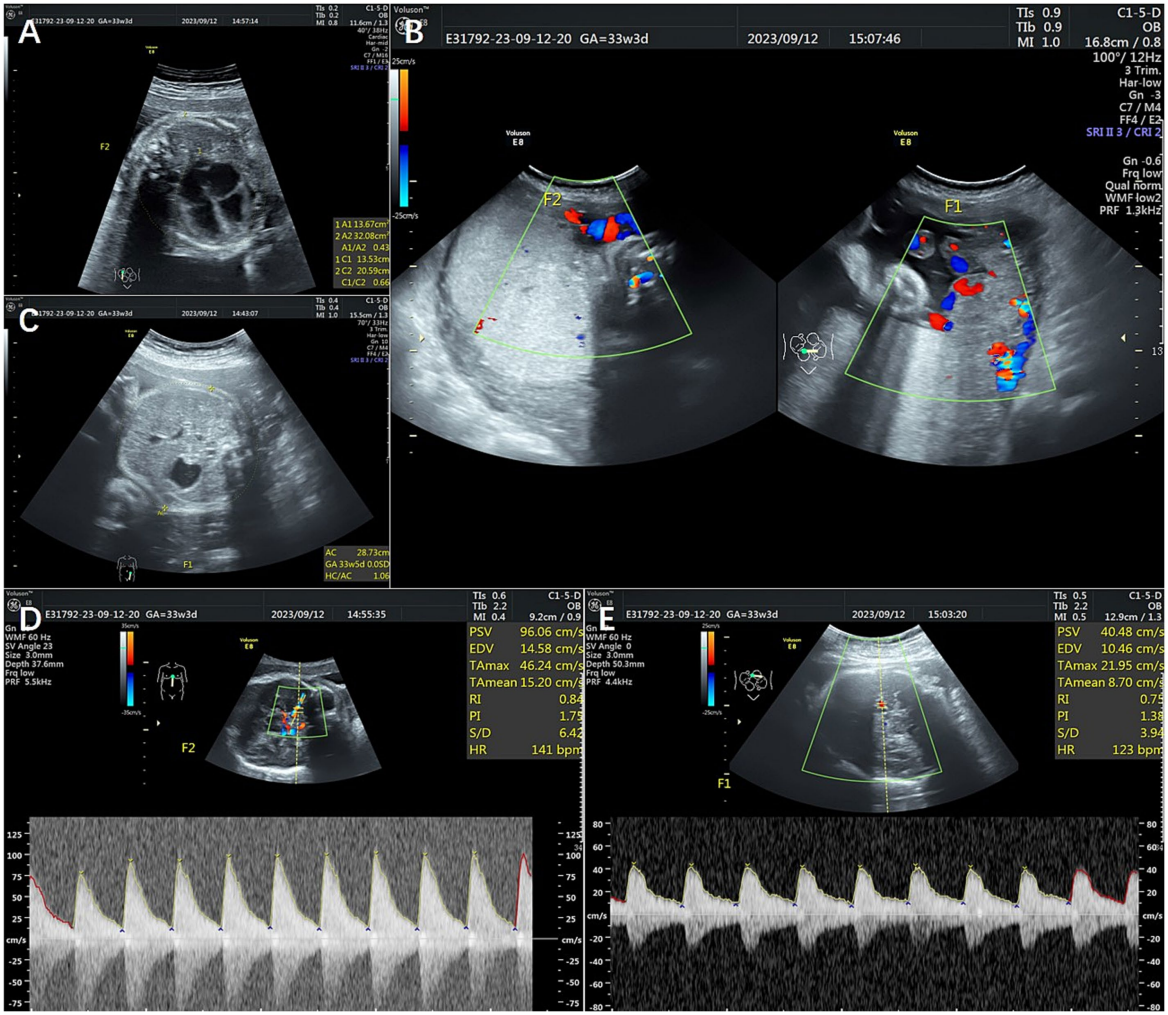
Ultrasonography of Patient 1. (A) Shows an increased cardiothoracic ratio of 0.66. (B) Shows the hyperechoic placenta in twin A and the thickened and hypoechoic placenta in twin B. (C) Shows a starry sky liver. (D,E) Show abnormal middle cerebral artery peak systolic velocities (MCA-PSVs) in both twins. F1 shows twin A, and F2 shows twin B.
